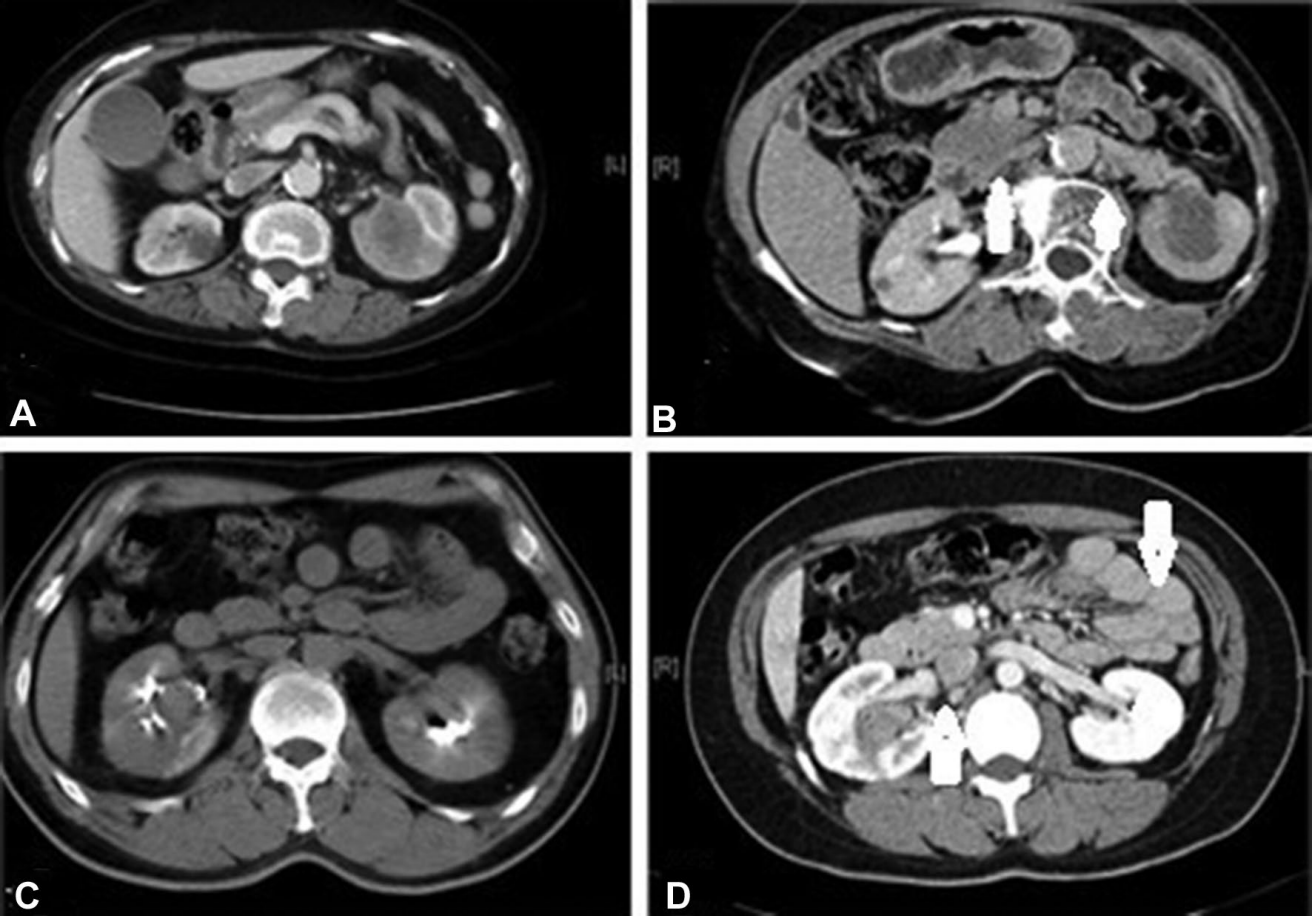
CT scans showing peritoneal thickening of renal pelvis carcinoma. Case 1: axial view of left renal pelvis malignancy showing a the absence of peritoneal thickening and b the presence of focal bowel wall thickening. Case 2: axial view of right renal pelvis malignancy showing c the absence of peritoneal thickening and d the presence of diffuse bowel wall thickening. Arrows indicate areas of peritoneal thickening
